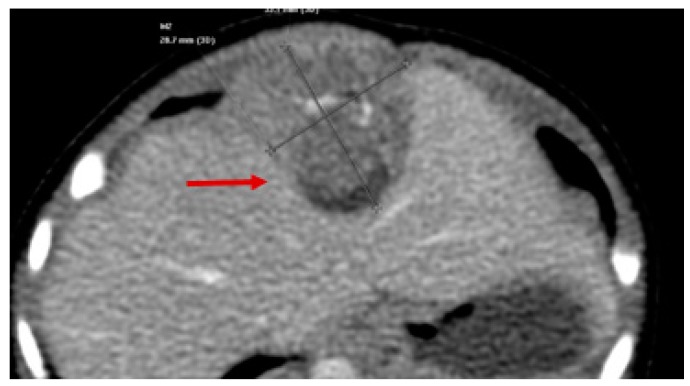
Axial CT showing Diaphragmatic Rhabdomyosarcoma (red arrow).
